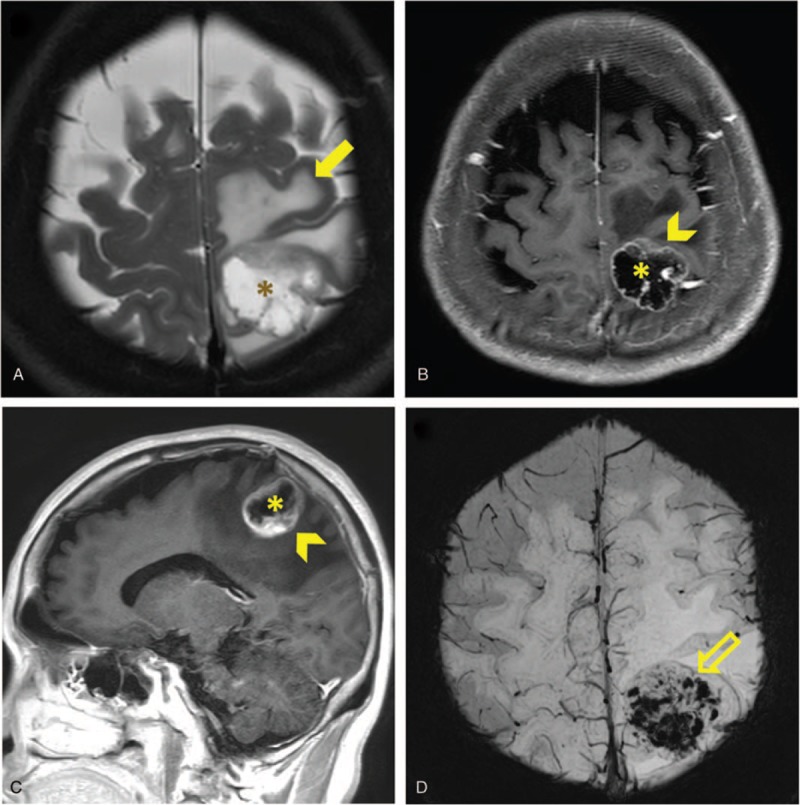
Representative MR images showing tumor necrosis, rim enhancement, peri-tumoral edema, and hemorrhage. A 66-year-old female NSCLC patient with left parietal metastasis. Axial nonenhanced T2-weighted MR images (A) shows central necrosis (asterisk) and peri-tumoral vasogenic edema (arrow). Axial (B) and sagittal (C) contrast-enhanced T1-weighted MR image shows central necrosis (asterisk) and rim enhancement (arrowhead). Axial susceptibility weighted image (D) shows hypointensities within the tumor (hollow arrow), suggesting hemorrhage.
